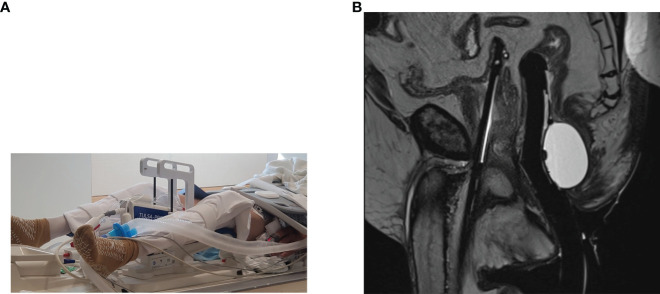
(A) Photograph of the TULSA-PRO device. (B) T2-weighted sagittal intraprocedural MRI demonstrating the TULSA-PRO device *in situ* within the urethra, as well as an intra-rectal balloon for cooling. (Images provided by Dr Sandeep Arora, Yale School of Medicine).
